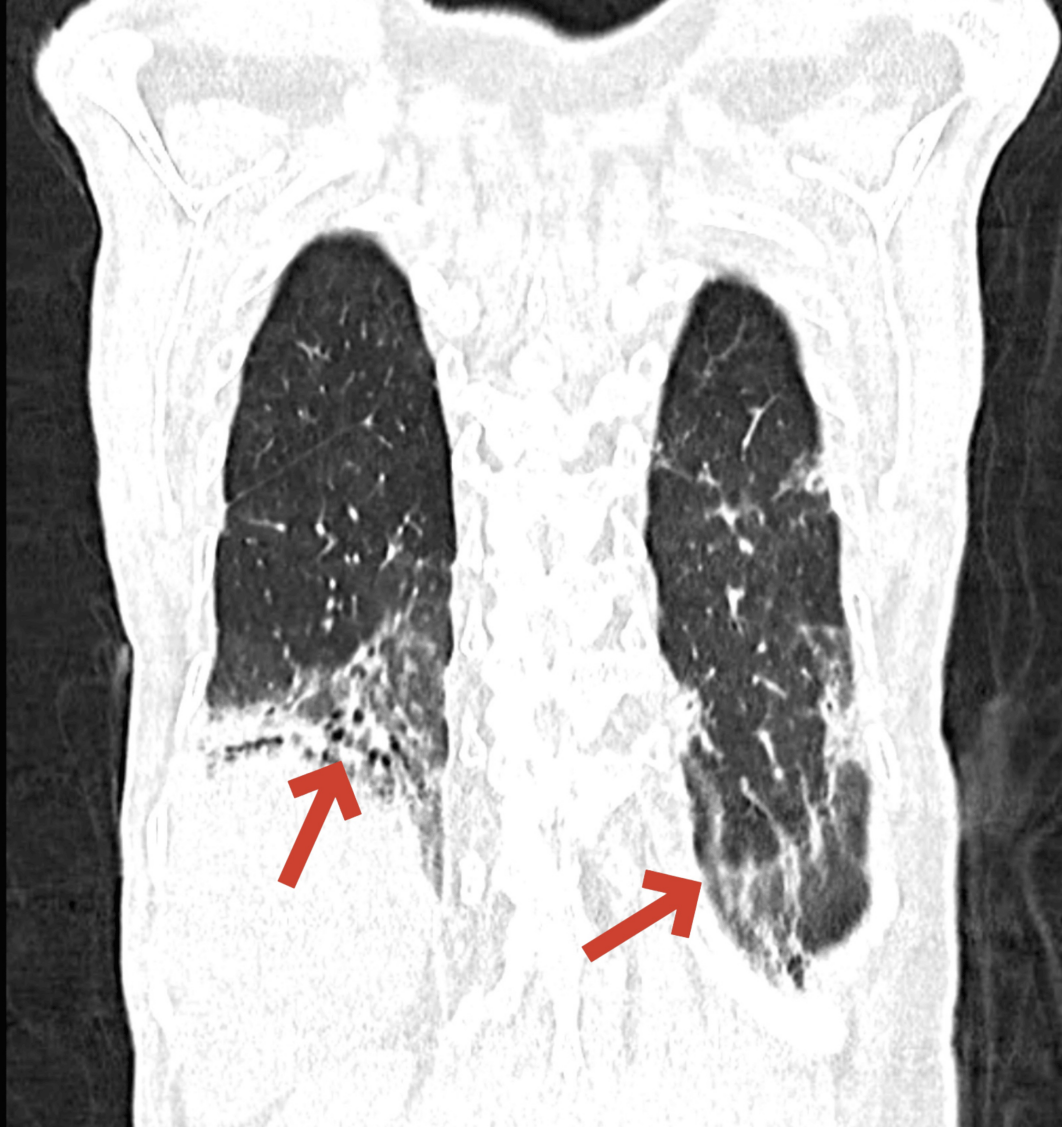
Axial CT thorax revealing diffuse lung pathology CT: Computed tomography The axial CT image reveals diffuse lung pathology with multifocal areas of ground-glass opacities, consolidatory changes, and tractional bronchiectasis as highlighted by the red arrows. These features suggest a progressive interstitial lung disease in the context of a systemic autoimmune disorder
